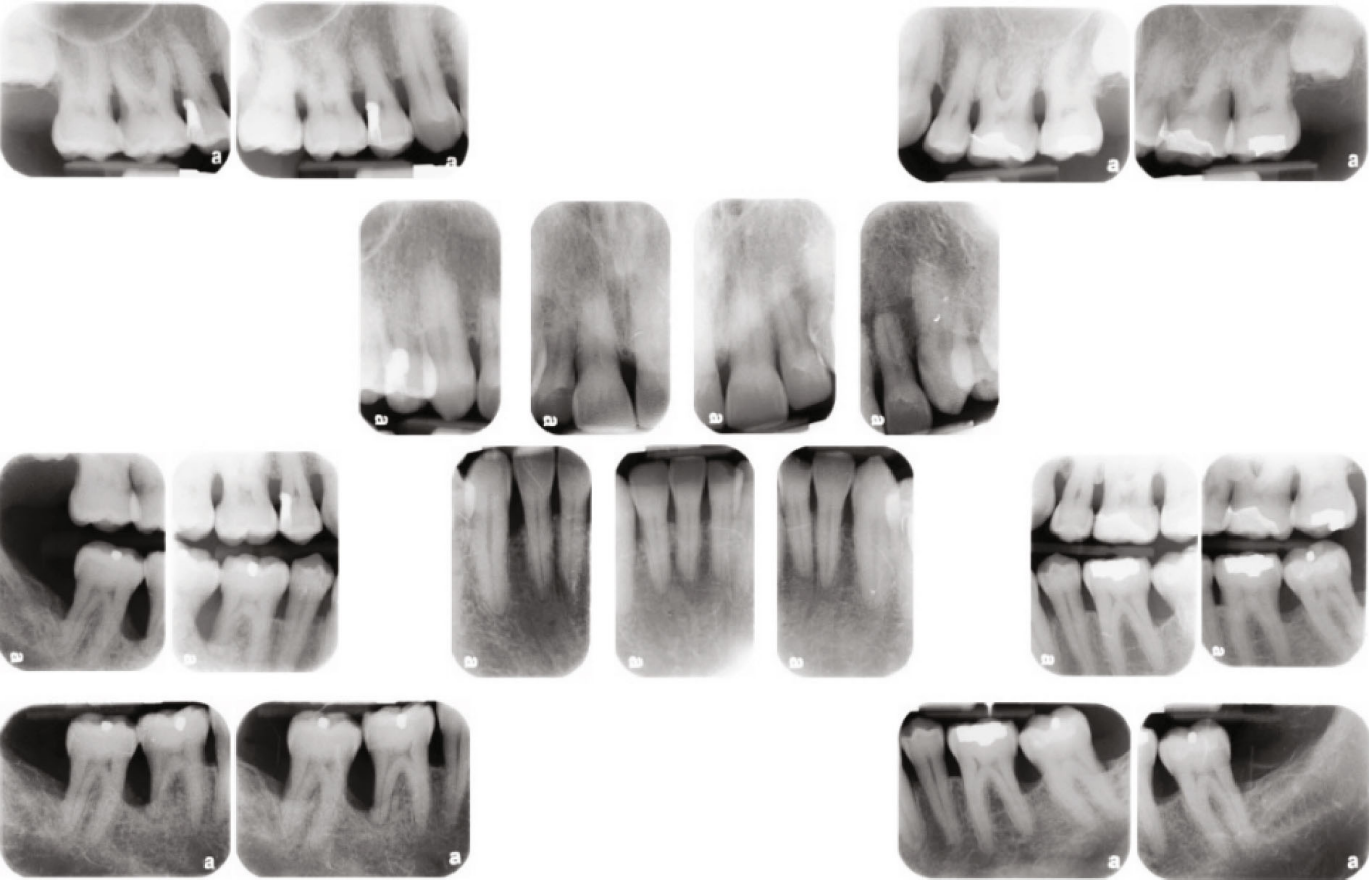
Full-mouth radiographic survey (FMRS) taken at the periodontal office, November 20, 2006.
